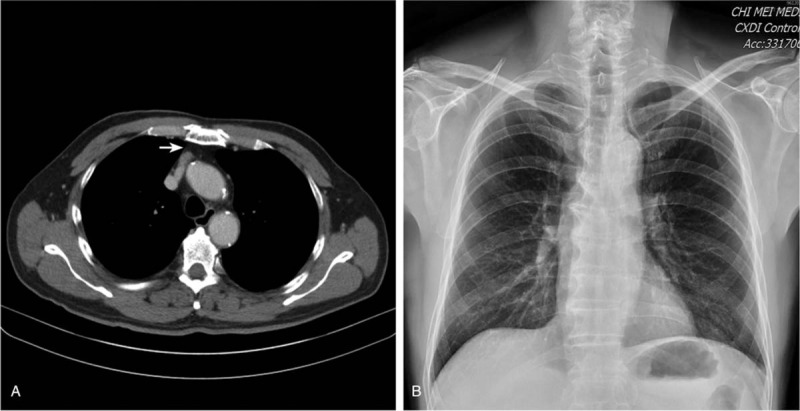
(A) Thymic hyperplasia on thoracic computed tomography (CT) (arrow); (B) Unremarkable finding on preoperative chest radiograph, indicating unlikely non-pulmonary origin of postoperative respiratory distress. CT = computed tomography.
